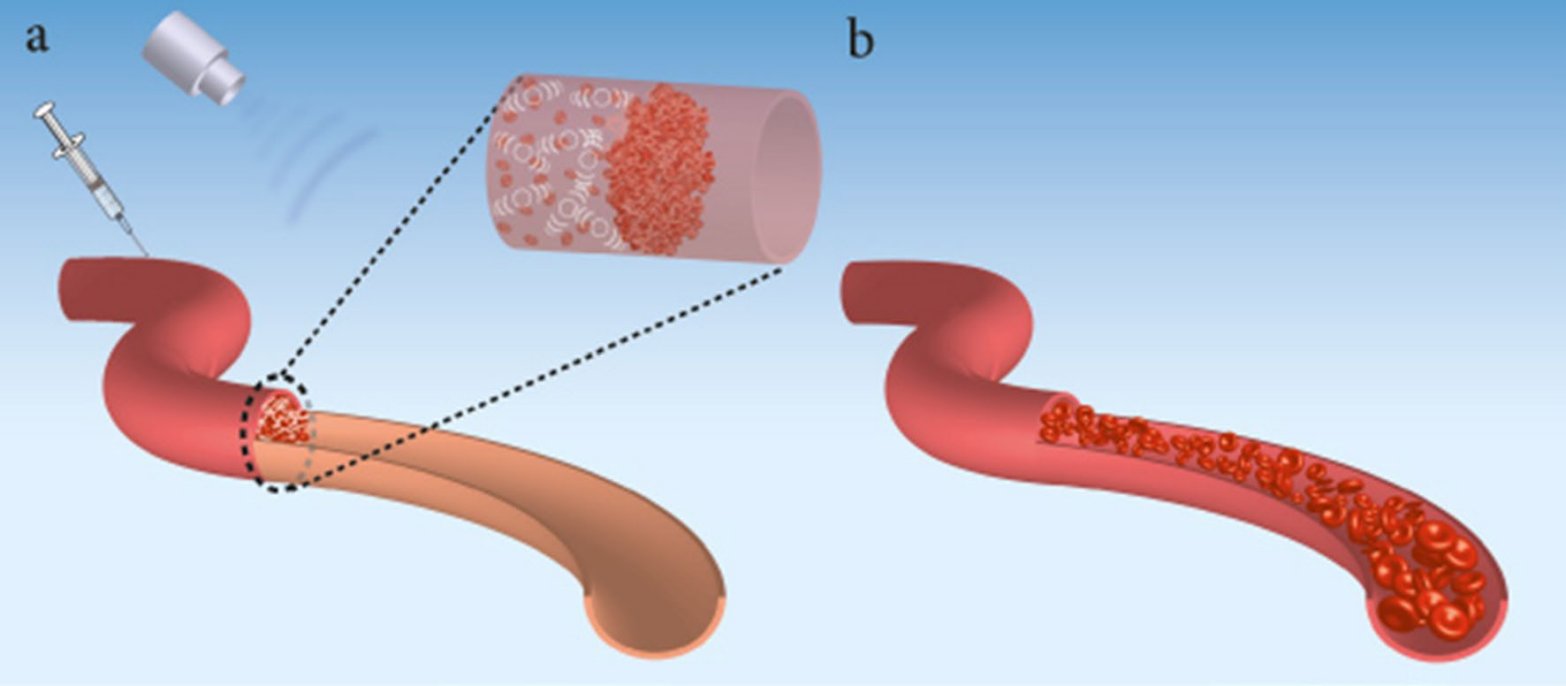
Improved sonothrombolysis of obstructive blood clots mediated with microbubbles. a Shows a blood vessel obstruction caused by fibrin clots, platelets, and RBCs. Microbubbles are injected (e.g. by intravenous infusion) and collected near the clot. b The US is used and cavitates the microbubbles that dissolve the blood clot and restore the flow. The mechanical bioeffects of ultrasonic-based microbubbles are used to enhance thrombolytic medications diffuse into blood clots and/or mechanically break down them. The commonly recognized thrombolysis progression concept is that the US-based microbubbles can generate inertial cavitation, micro-streaming, acoustic radiation, and steady cavitation force to temporally “loosen” clots of fibrin and promote thrombolytic medication distribution/diffusion, leading to faster and more precise clot treatment. Also, using the US-based microbubbles strategy, the degree of force generated and, as a consequence, the degree of clot displacement is controlled by adjusting the transducer's acoustic intensity and center frequency
